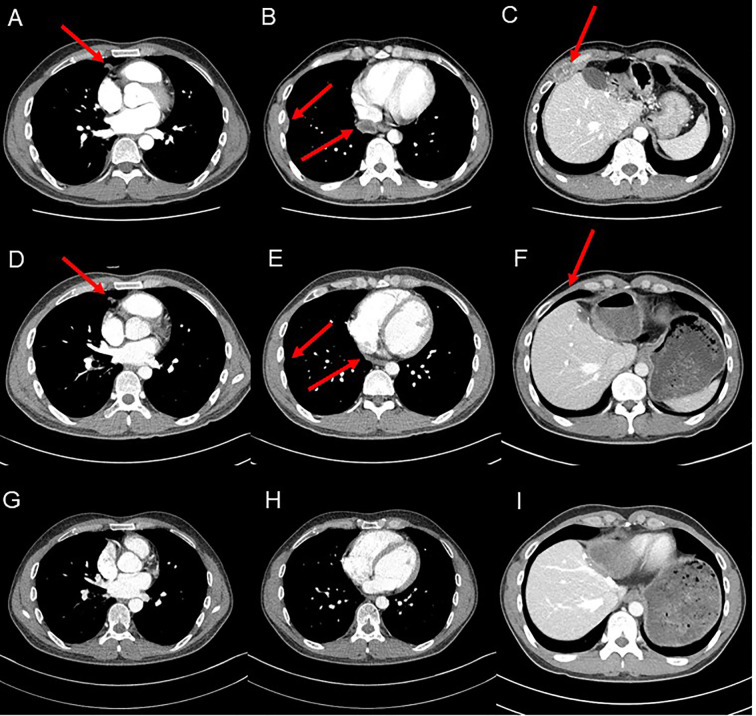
Computed tomography (CT) imaging. CT images showing treatment response to nivolumab. (A–C) Baseline CT reveals ovoid hypodense masses (<3.0 cm) at the retrocaval area, right anterior chest wall, and right lateral pleura. (D–F) After 9 weeks of nivolumab, the masses show marked size reduction. (G–I) After 33 weeks, no definite metastatic lesions are observed in the previously involved sites.
